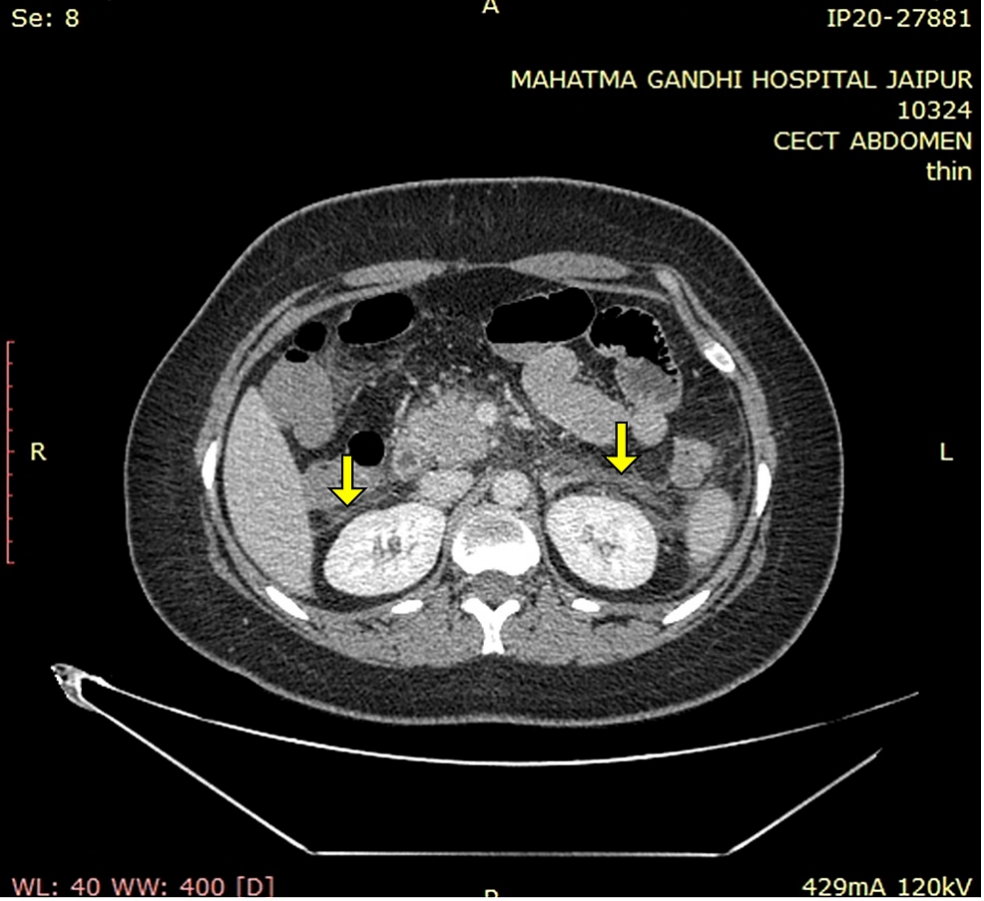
Contrast-enhanced CT of the abdomen (axial sections) showing bilateral renal and lateroconal fascial thickening (solid yellow arrows).
